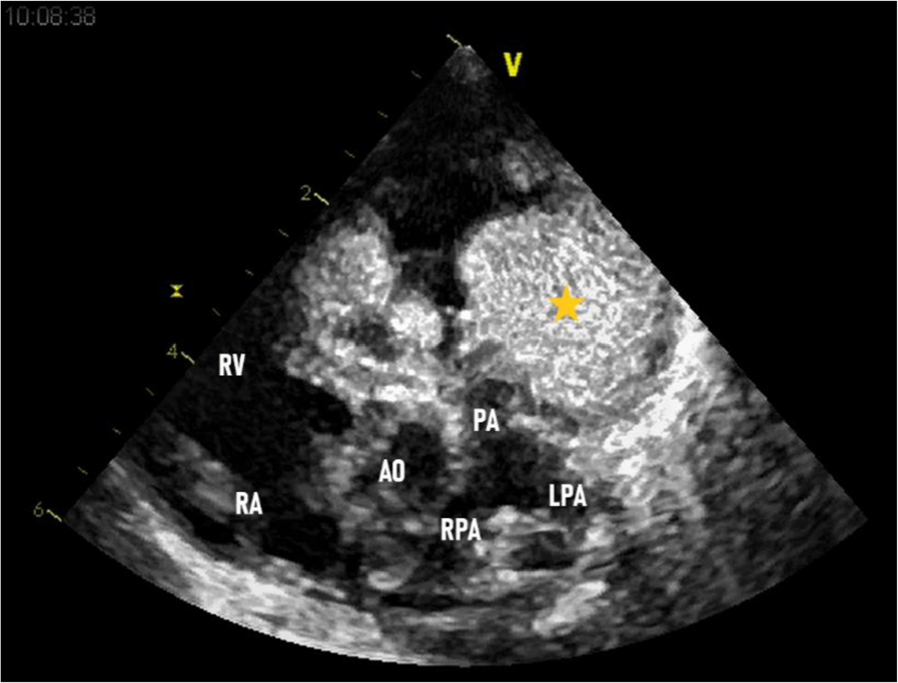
Parasternal short axis view at the level of the aortic valve. Asterisk showing the obstructive mass in the RVOT. pulmonary artery (PA), right pulmonary artery (RPA) and left pulmonary artery (LPA), right ventricle outflow tract (RVOT)
